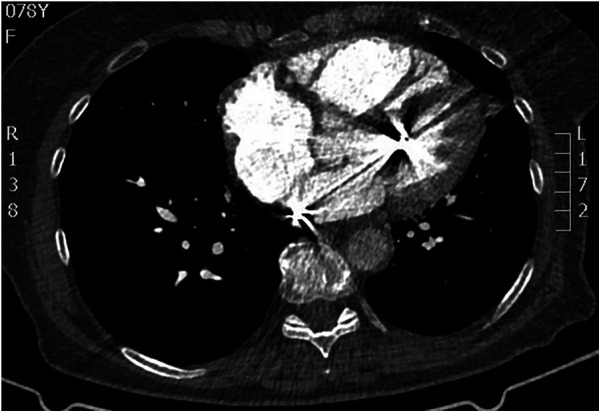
CT scan demonstrating malpositioned cardiac pacing leads located within the left heart.
